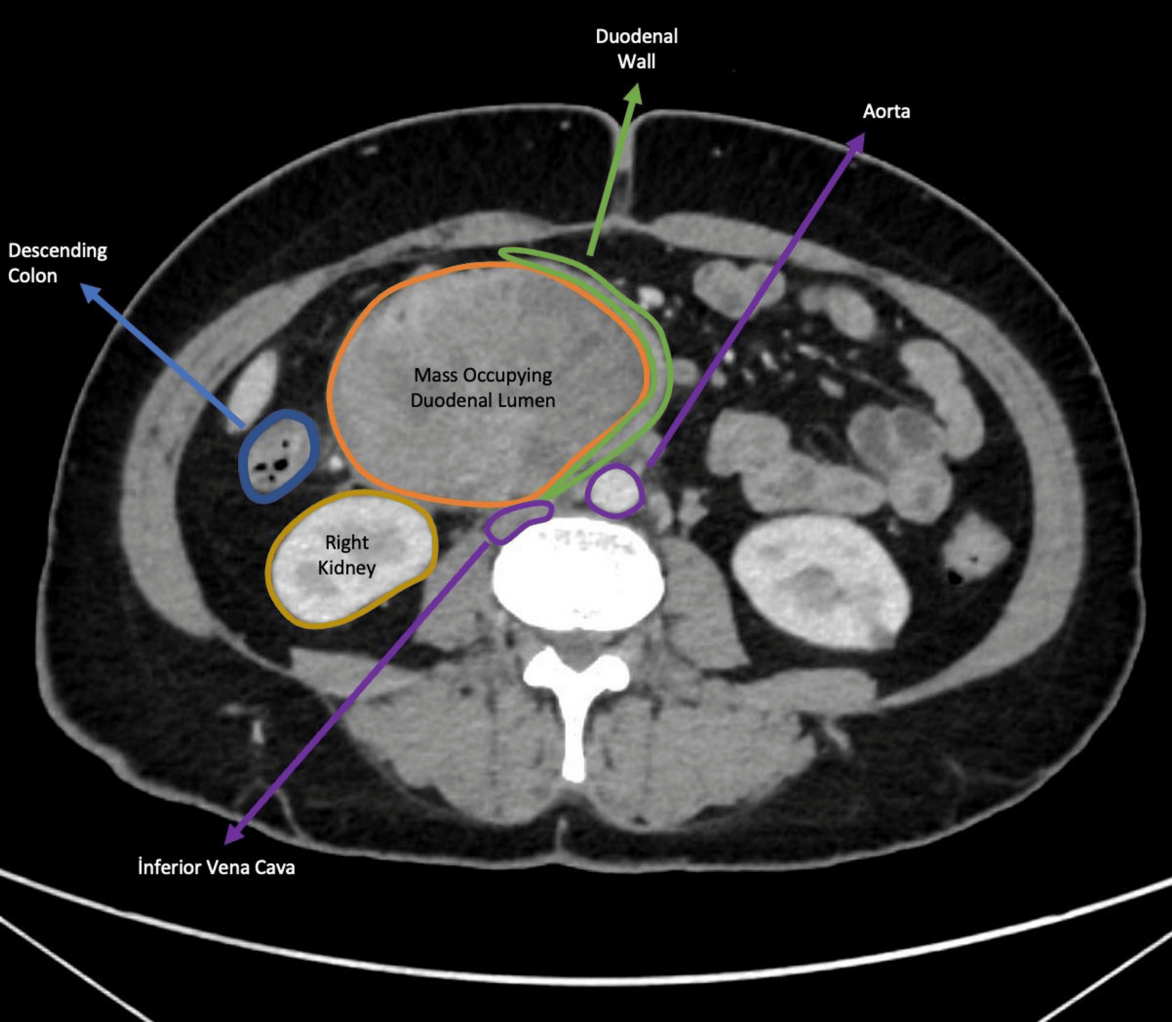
Post-contrast axial CT image demonstrating an intraluminal duodenal mass. A heterogeneously enhancing solid mass is seen within the duodenal lumen. No component extends beyond the lumen.
